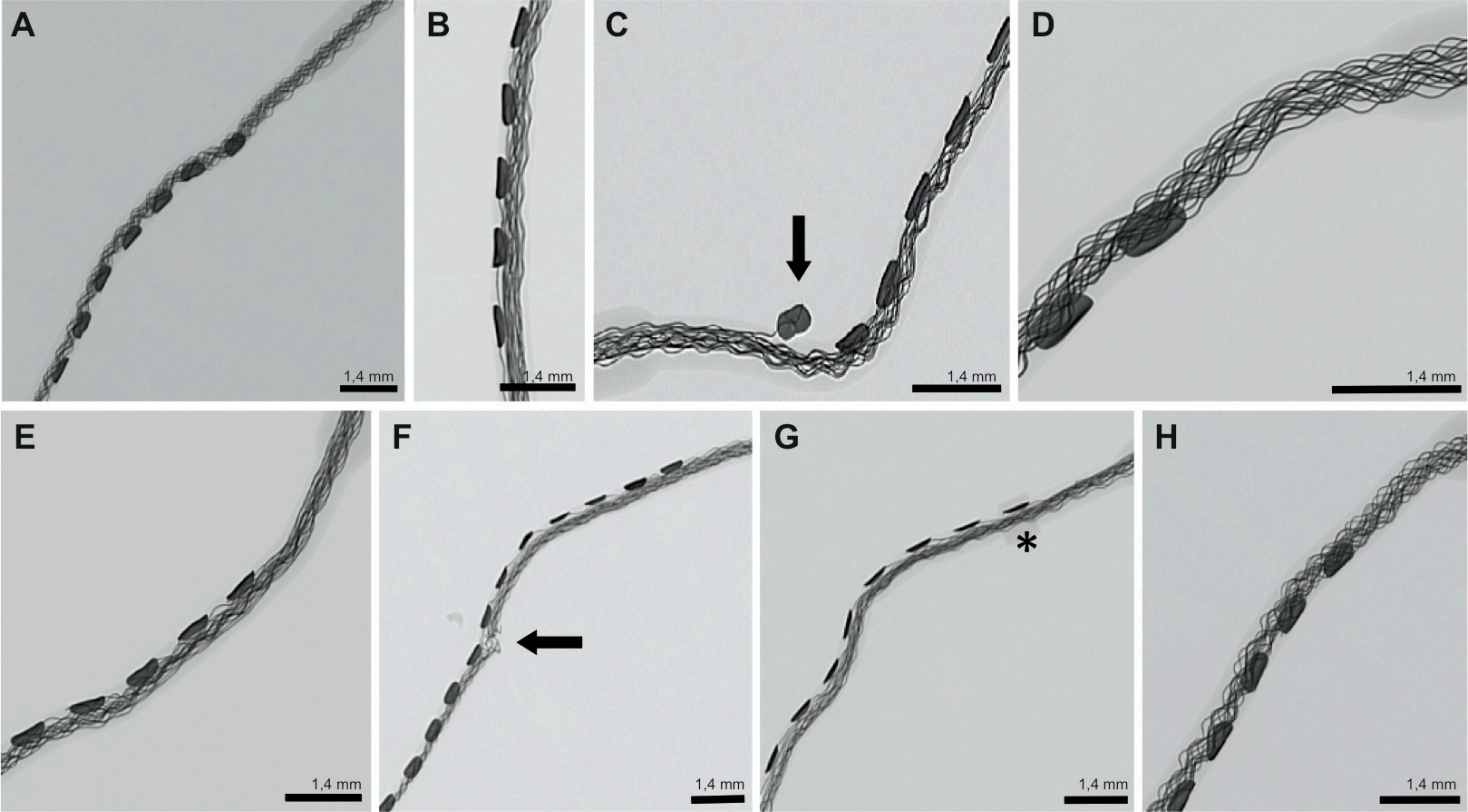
Micro-X-ray of the electrode-Prototypes 3–10 after removal; the arrows marking the wire-damage of electrode 5 and 8. 5A: Prototype 3: no relevant damage, slight kinking at the very base; 5B: Prototype 4: no damage, no kinking; 5C: Prototype 5: showing a very high kinking and the detached contact at the base at Prototype 5; 5D: Prototype 6: slight kinking at the base; 5E: Prototype 7: slight kinking at the base; 5 F: Prototype 8: the electrode array of Prototype 8 was cut; 5 G: Prototype 9: the last insulation ring of Prototype 9 is still in place—Asterisk); 5 H: Prototype 10: no damage, no kinking.
